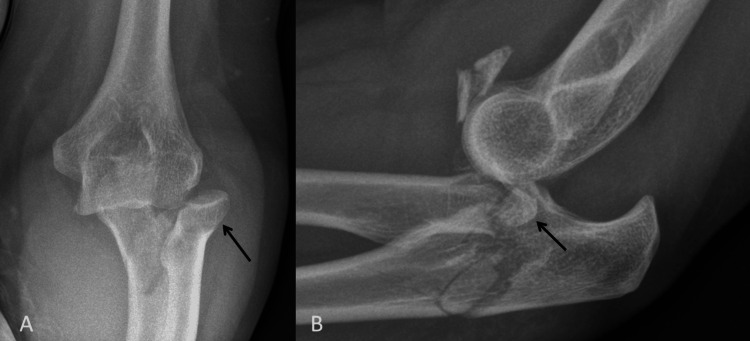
Pre-operative radiographs demonstrating a fracture/dislocation of the elbow. A: Antero-posterior view showing dislocated radial head (black arrow), which is also fractured. B: Lateral view demonstrating significant comminution of radial head (back arrow) and also, a comminuted fracture of the ulna.
